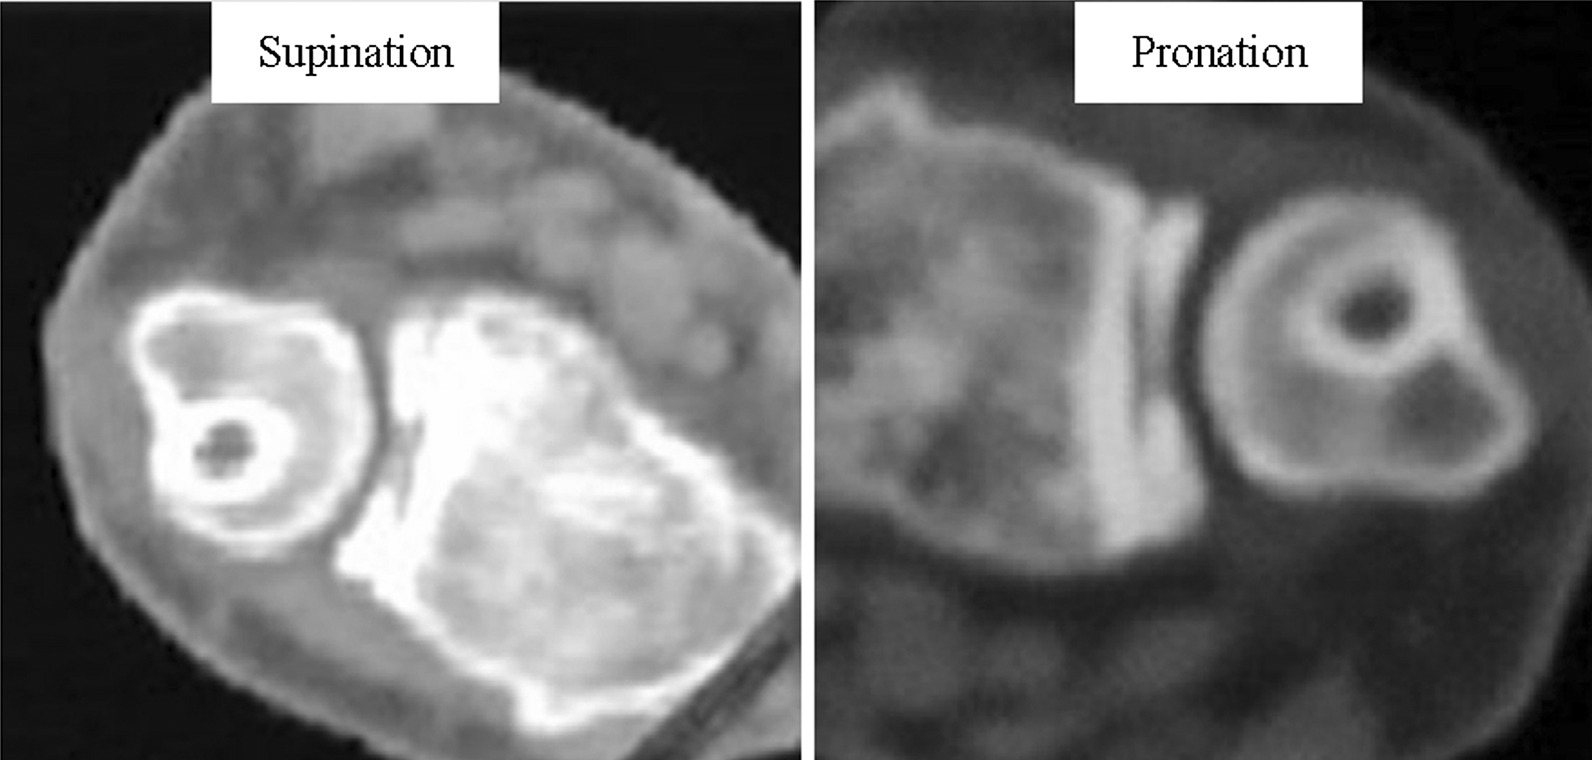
Postoperative computed tomography of wrist in supine and prone position. The supine and prone positions of the wrist demonstrate good distal radioulnar joint congruency and no motion limitation
